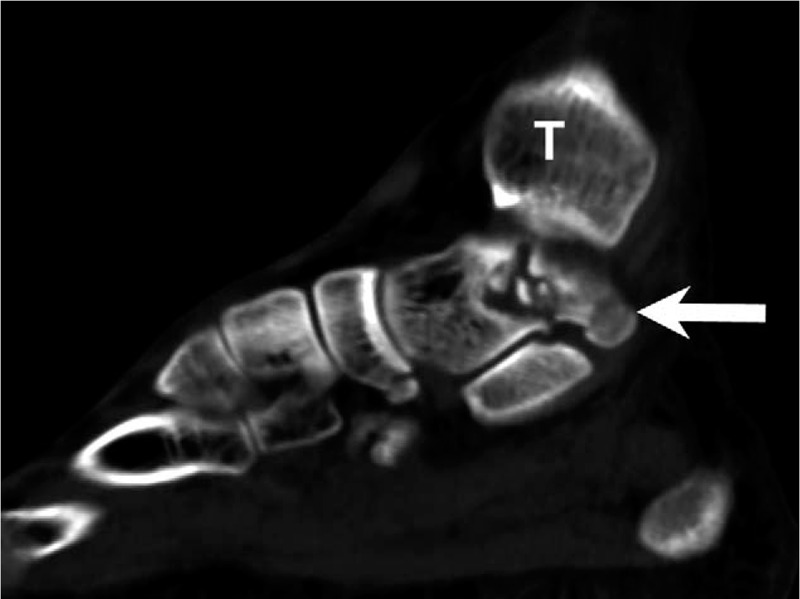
In congruity examination, computed tomography was used to diagnose talar neck fracture. Arrow = talus, T = tibia.
